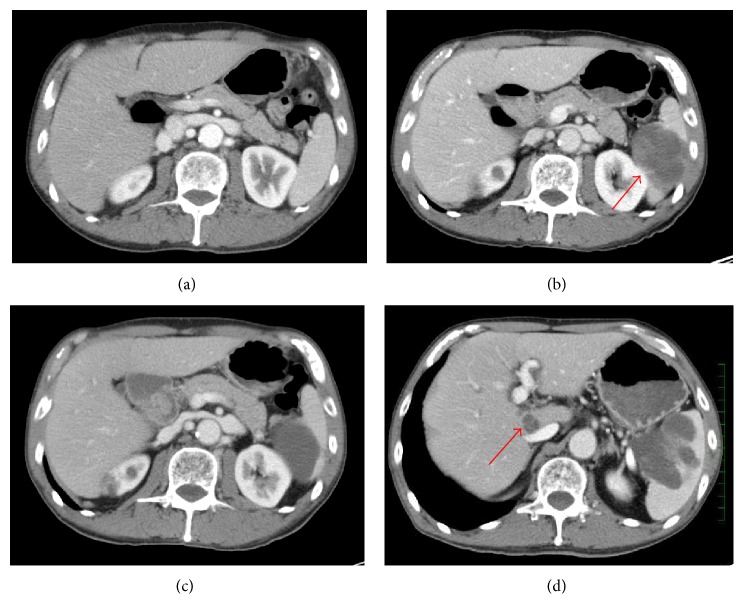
(a) There are no abnormal findings in abdominal CT scan before surgery. (b) Abdominal CT scan with contrast 6 weeks after surgery shows a mass with inhomogeneous opacity in the spleen (arrow). (c) Abdominal CT scan with contrast after the 3 courses of chemotherapy by carboplatin and paclitaxel plus bevacizumab. The tumor size is reduced and its inhomogeneous opacity changed to homogeneous opacity. (d) Abdominal CT scan with contrast after the 4 courses of chemotherapy shows a new metastasis to the liver and enlarged metastatic tumor in the spleen (arrow).
